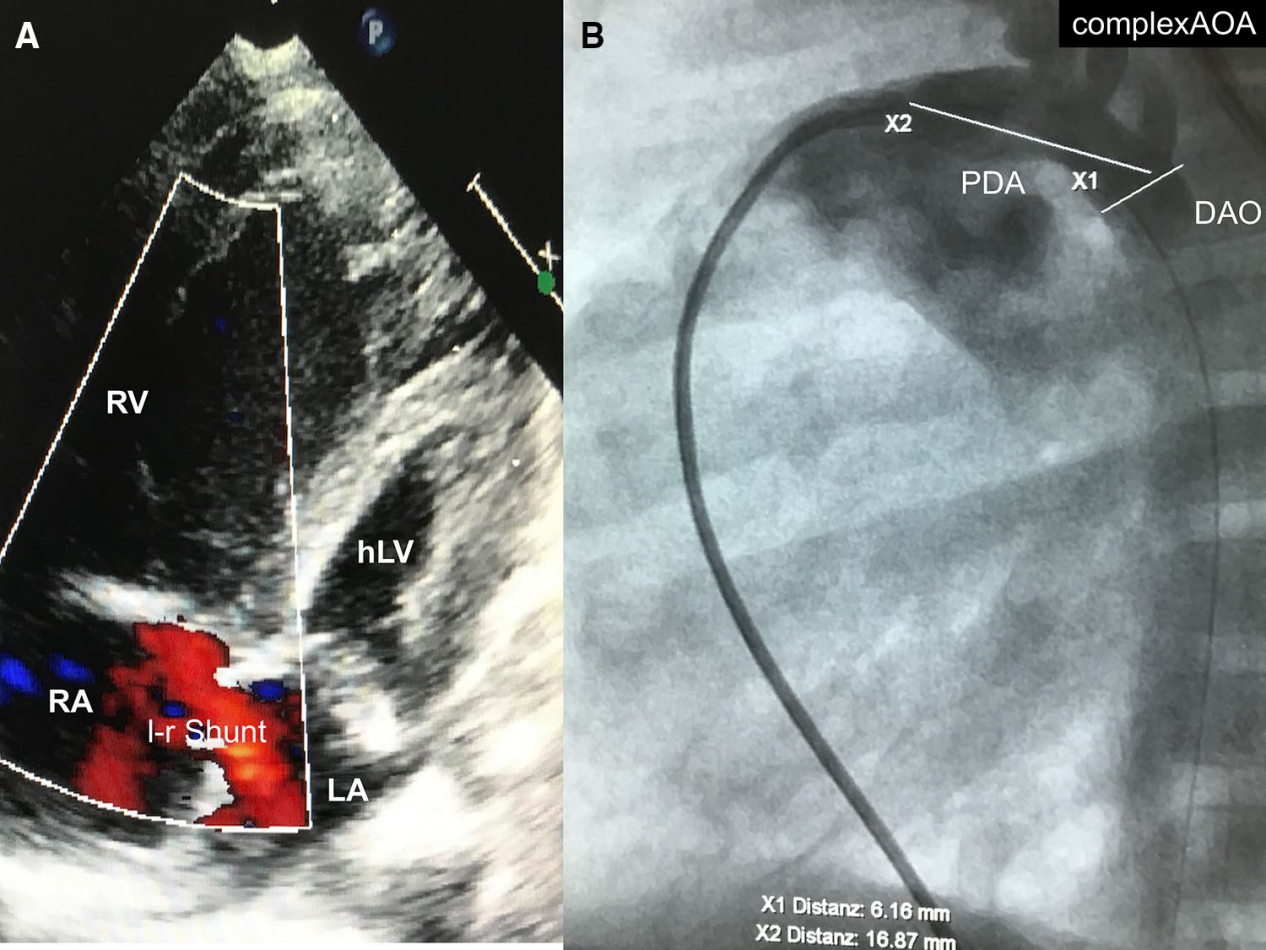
a, b Echocardiographic four-chamber view of a patient with HLHS (hypoplastic left heart syndrome) demonstrates left–right shunt across a non-restrictive atrial communication (a); lateral 90° angiography of right-left shunting, non-restrictive patent arterial duct (PDA) connected pulmonary artery to descending aorta (DAO) in a complex aortic arch (AoA) without clear differentiation of duct and aortic arch (b). *hLV* hypoplastic left ventricle, *LA* left atrium, *RA* right atrium, *RV* right ventricle
